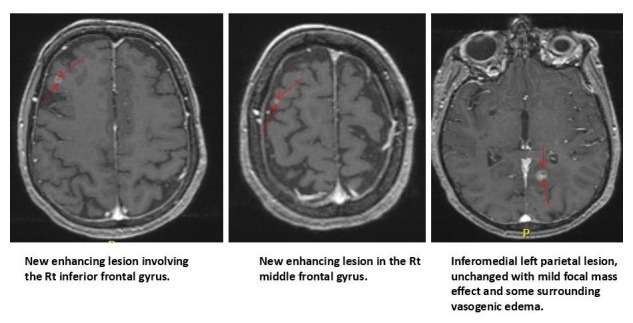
Follow-up MRI brain with new metastases and old lesion. MRI: Magnetic resonance imaging.
